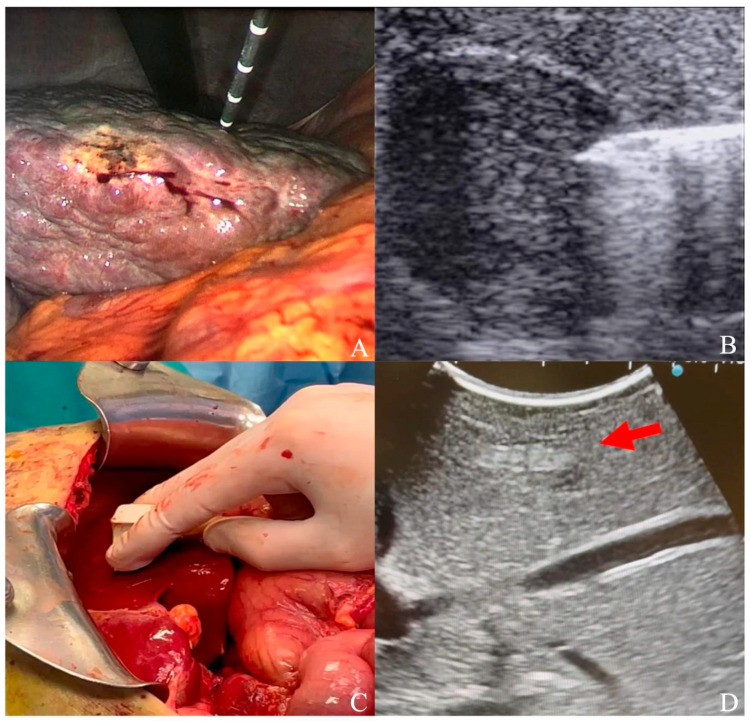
(A) Laparoscopic ultrasound-guided thermal ablation with microwave antenna; (B) microwave antenna approaching a hypoechoic HCC under laparoscopic ultrasound guidance; (C) laparotomic ultrasound; (D) hyperechoic HCC (red arrow) visualized under liver surface.
